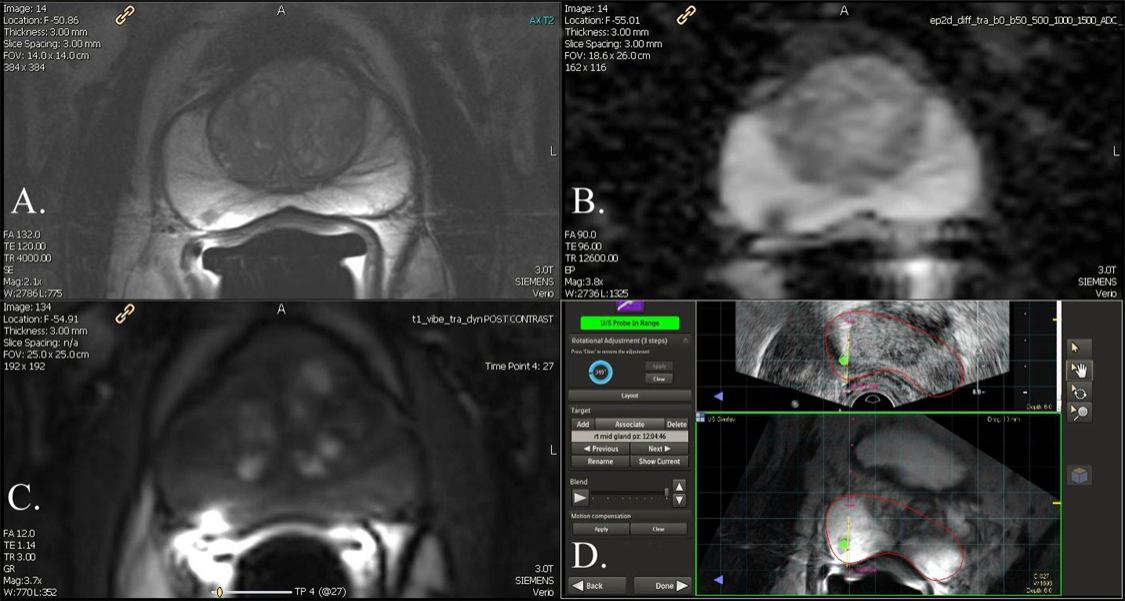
Prostate mpMRI. A. T2 sequence with a low signal intensity in Zone 3L. B. DWI with corresponding ADC restriction C. DCE with early arterial uptake. D. MR US fusion directed biopsy detected Gleason 3+4, 2.5 mm. Please see supplemental report regarding specific lesion based reporting.
